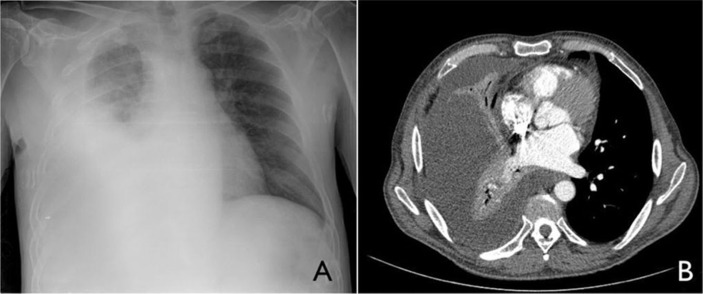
Initial chest radiograph (A) and CT (B) showing a large loculated right-sided pleural effusion.
